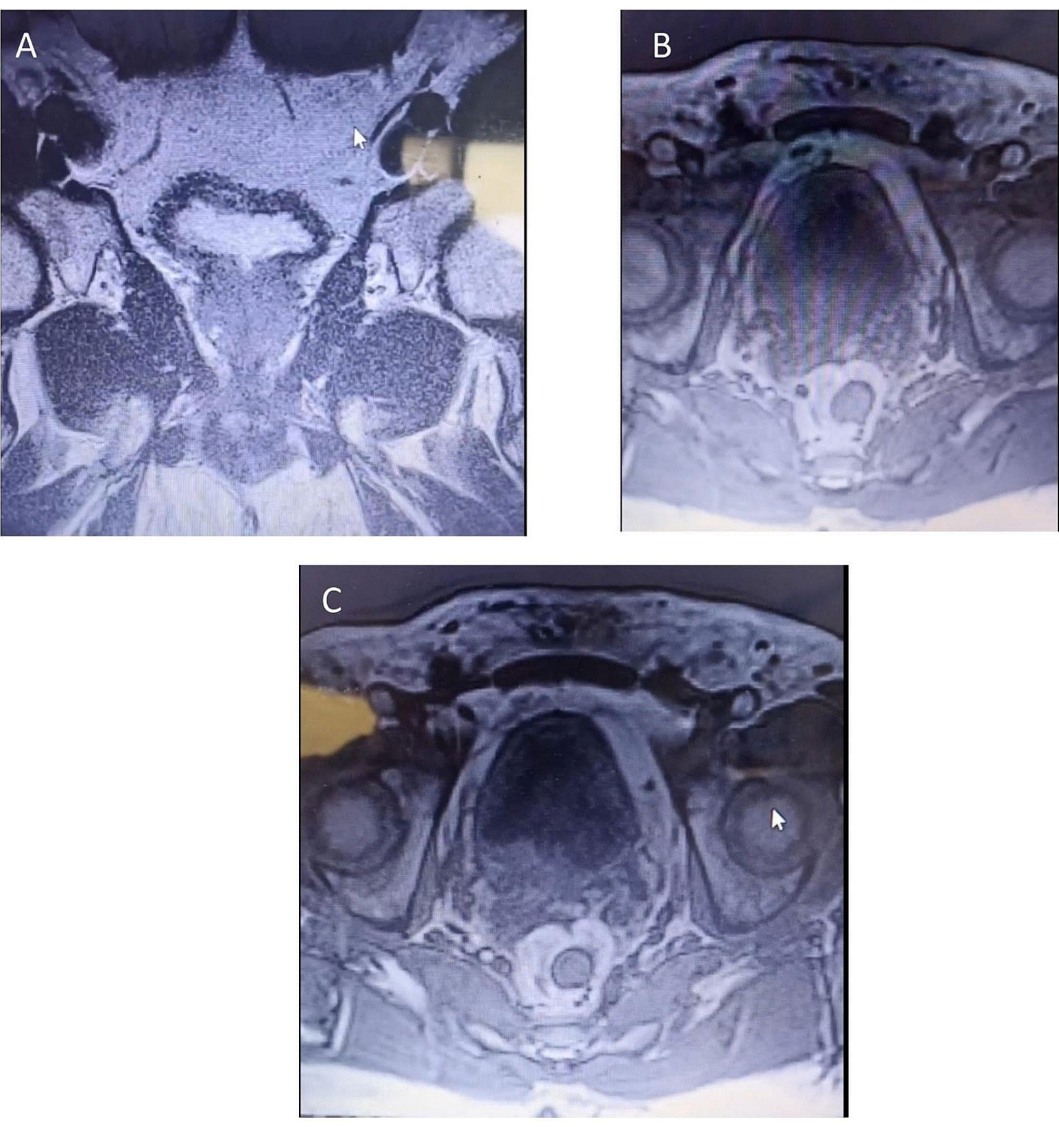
MRI revealed (A) a lesion exhibiting high signal intensity on T1-weighted images within the right peripheral zone of the prostate. This finding suggests the presence of a possible tumor or hemorrhage, which could be a post-biopsy complication. (B, C) A slight thickening of the bladder wall mucosa was observed post-injection, suggesting potential inflammatory or neoplastic alterations
